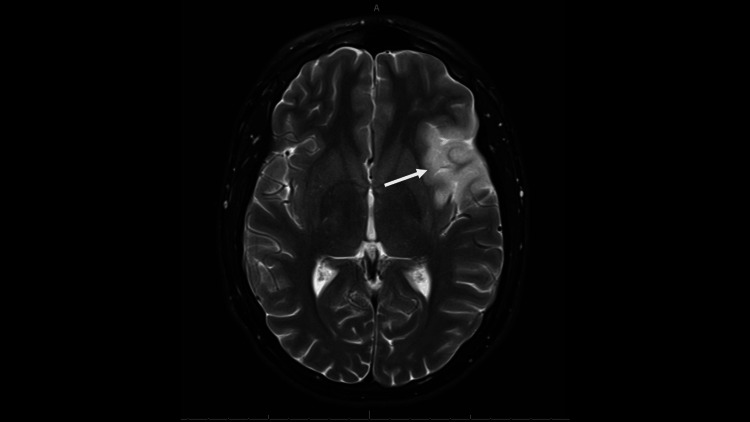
Axial, diffuse weighted image sequences from MRI of the brain Arrow demonstrates increased signal intensity, demonstrating evolving left MCA-territory ischemic infarction MCA: middle cerebral artery; MRI: magnetic resonance imaging
